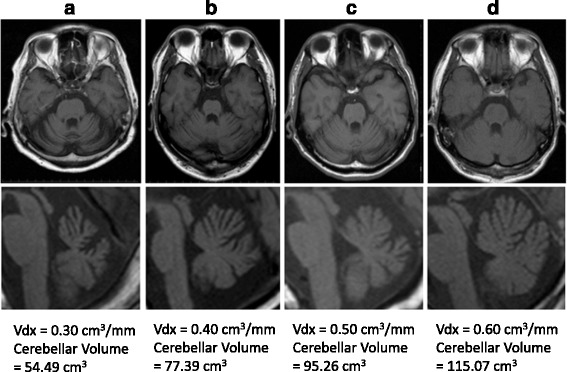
Representative images of cerebellar atrophy (T1-weighted image) and Vdx (a-d). Vdx is the cerebellar volume index (Vdx = cerebellar volume/cranial AP diameter). The mean Vdx value in normal adults (mean age, 64.2 ± 18.7 years; cranial AP diameter, 18.2 ± 1.1 cm) is 0.65 ± 0.06 cm^3^/mm. Vdx can be used to quantify the decrease in volume due to cerebellar atrophy, which is difficult to evaluate with visual qualitative assessment alone
